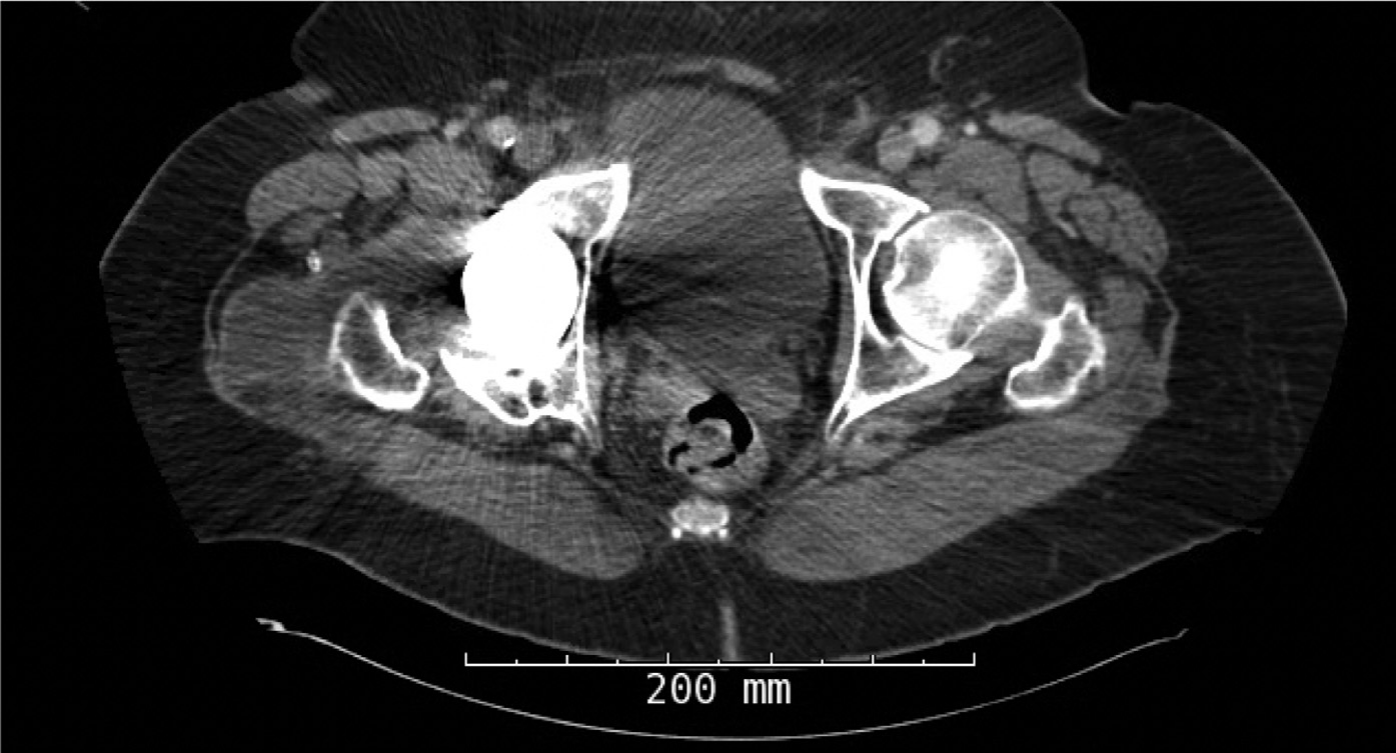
Axial CT scan demonstrating periprosthetic fluid collection.
